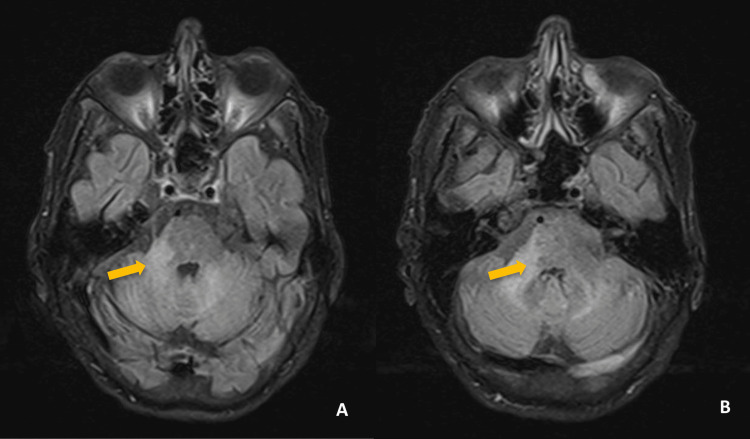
Third brain MRI performed three weeks after the previous one, transverse T2/FLAIR sequence (A and B), with a new lesion on the right brainstem (yellow arrows). FLAIR: fluid attenuated inversion recovery
